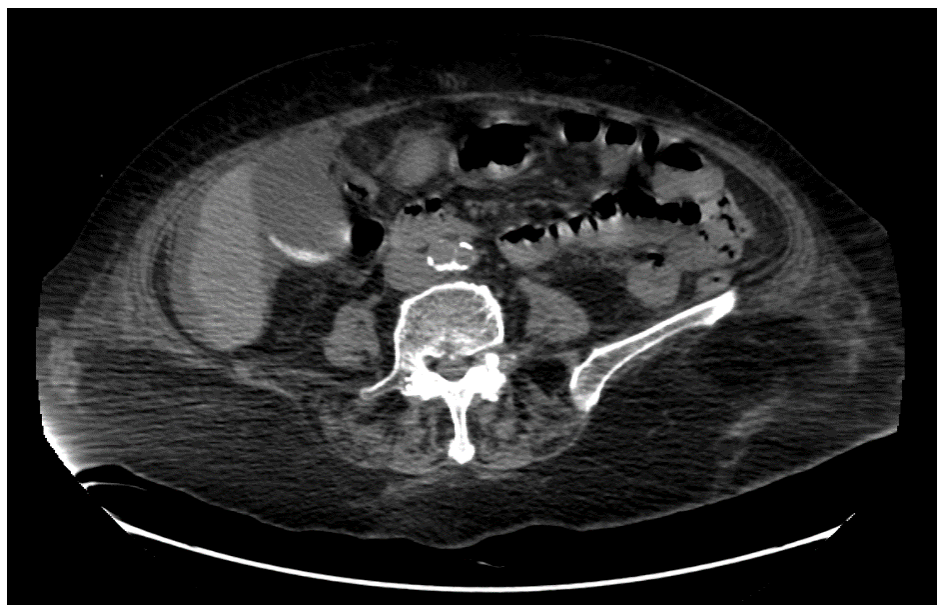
CT-scan showing a diffuse thickening of the gallbladder and inflammatory pericholecystic fluid.
